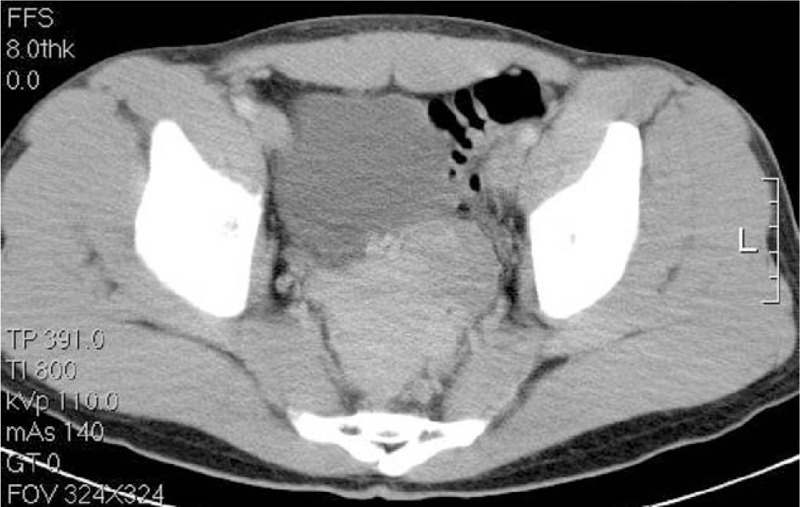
Abdominal computed tomography scan, horizontal view, revealing an 8.9 × 7.2 cm heterogeneous mass with a low-density area and wall thickening, suggesting a gastrointestinal stromal tumor.
